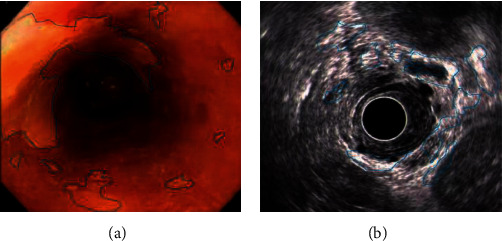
Ultrasonic image characteristics and endoscopy in patients with early upper gastrointestinal cancer. (a) Endoscopy in patients with early upper gastrointestinal cancer. (b) Ultrasonic image characteristics of patients with early upper gastrointestinal cancer.
